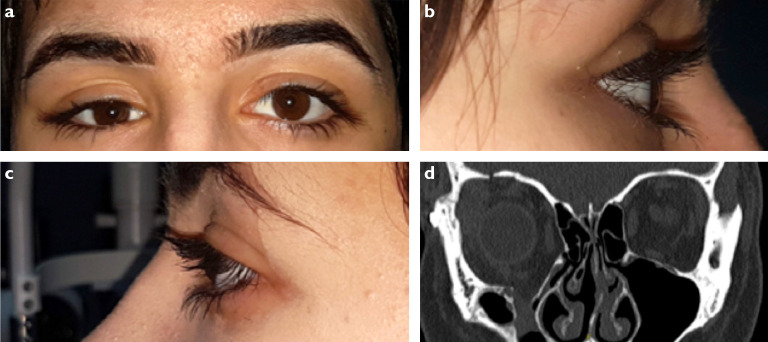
The clinical examination performed seven months after the initial surgery showing the (a) hypoblobus and enophthalmos, (b) right eye, (c) left eye (d) CT coronal scan with the floor and medial wall fracture.
